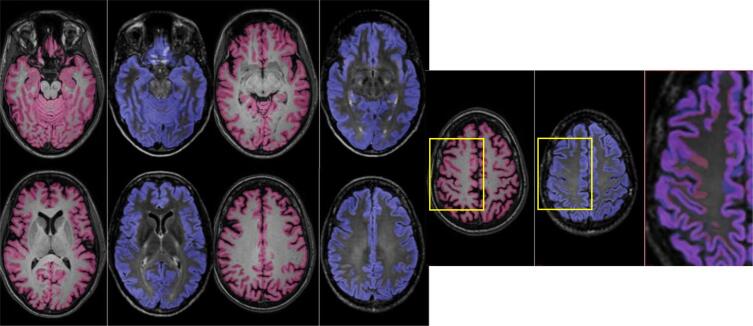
A subject’s cortical GM segmentation shown for 3T, using the multimodal GIF database. T1 segmentation is denoted in pink, and T2-FLAIR segmentation is shown in blue. An enlarged image overlaying both T2-FLAIR and T1 segmentations is included on the right of each series, showing areas of discrepancy, highlighted in the yellow boxes.
